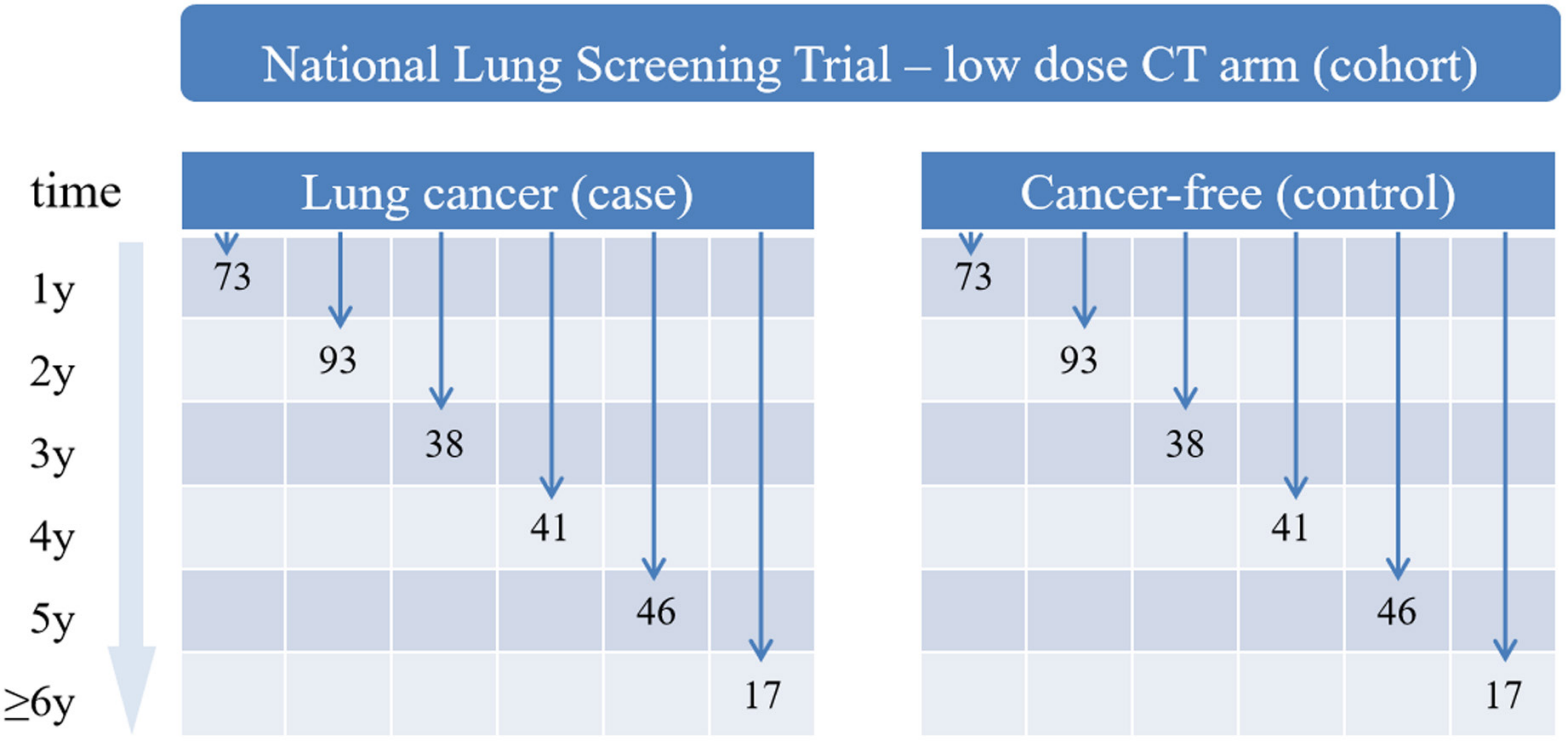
Nested case–control design. CT, computed tomography.
